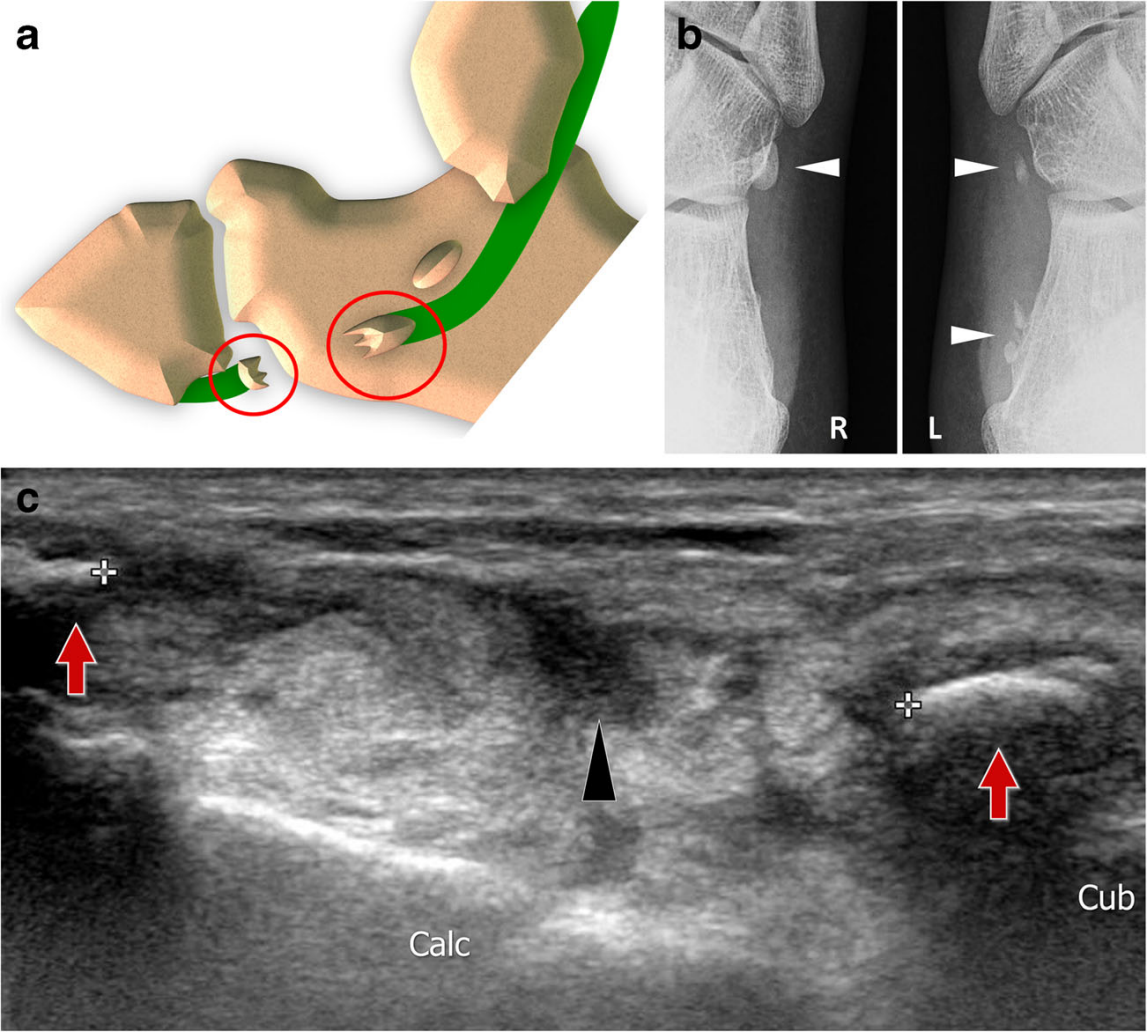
PLT tear type II. *13A* Scheme *13B* Standard internal oblique radiographs. On the left side, a lesion at the level of the OP with separation between fragments (*arrows*) of more than 6 mm. On the right side, the contralateral normal OP (*arrow*). *13C* Ultrasound. US clearly shows the two fragments (*arrows*) and the hematoma between them (*arrowhead*)
